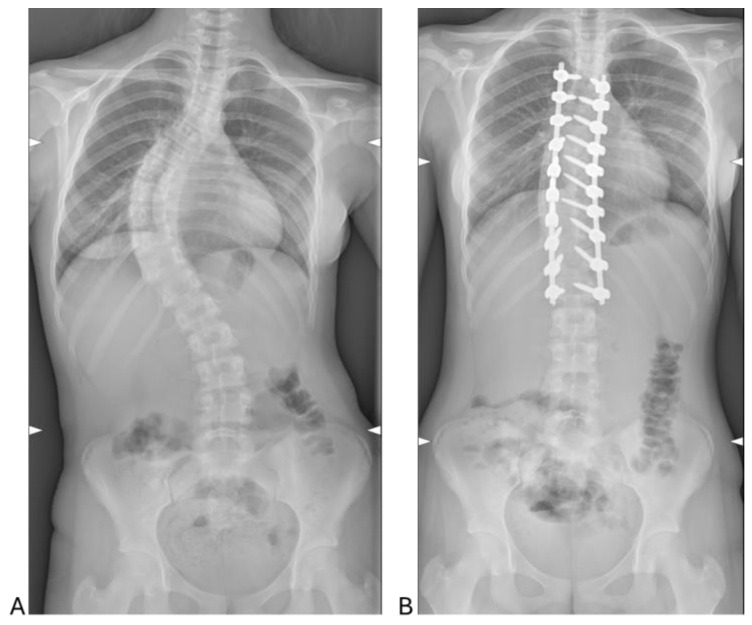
Pre- (A) and postoperative (B) radiographs of representative case with 13-year-old female patient. (A) Preoperative standing Cobb angle of 64° and (B) postoperative standing Cobb angle of 14°.
